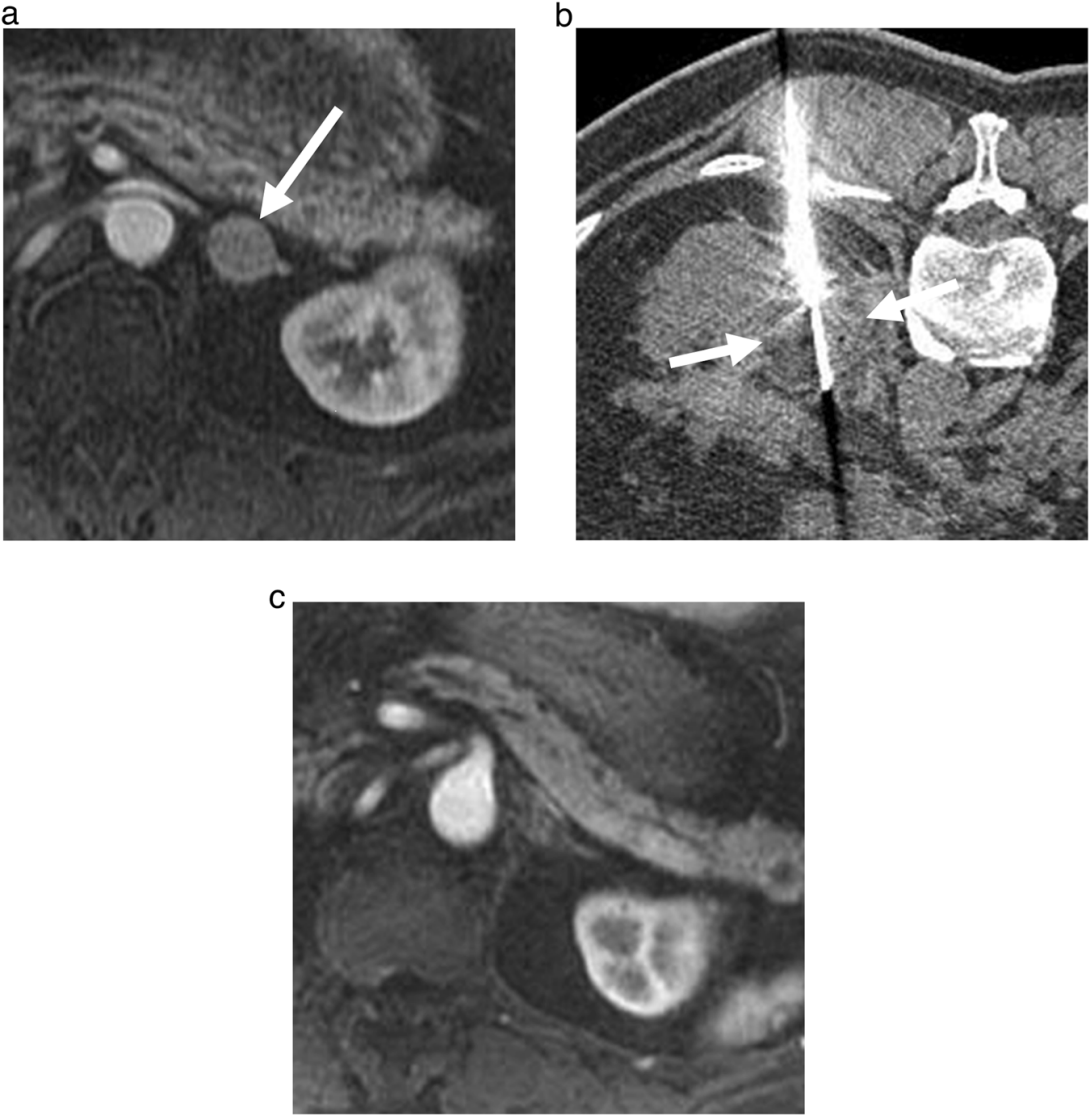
a Contrast-enhanced T1 weighted axial MRI image shows a 2 cm left adrenal metastasis from hepatocellular carcinoma (arrow); b CT image obtained during CA of the left adrenal metastasis with the patient in the prone position, with arrows showing iceball margin; and (c) contrast-enhanced T1 weighted axial MRI image 4 years following CA shows no residual or recurrent tumor
